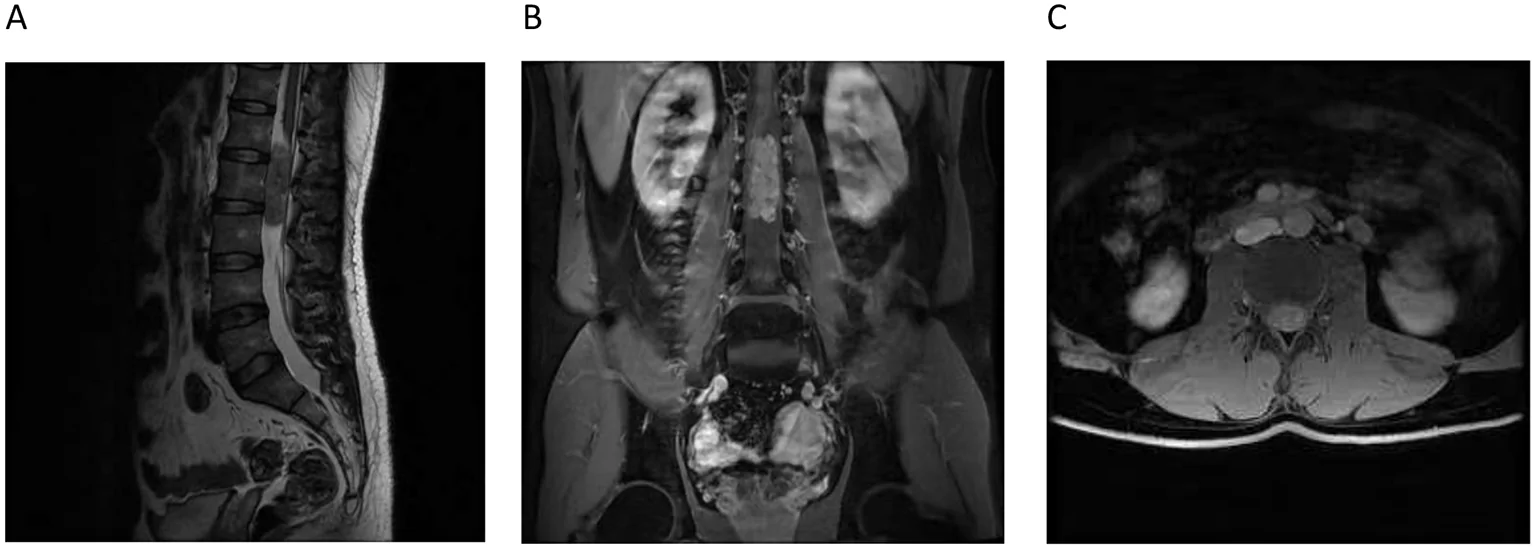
Lumbar spinal MRI findings in the patient. (A) Contrast-enhanced sagittal view; (B) Contrast-enhanced coronal view; (C) Contrast-enhanced axial view. A fusiform intradural tumor, measuring approximately 15 mm × 18 mm × 60 mm, is identified within the spinal canal at the L1–L3 vertebral levels. The lesion exhibits heterogeneous contrast enhancement. The cauda equina is compressed and displaced to the right; additionally, multiple tortuous vessels are observed adjacent to the spinal cord above the lesion.
